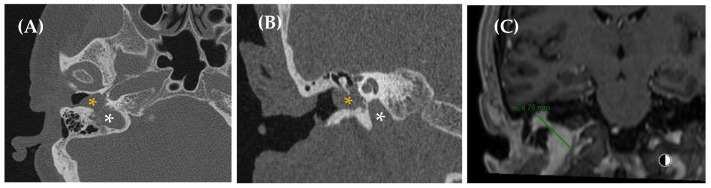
A jugulotympanic paraganglioma in a 60-year-old woman presented with somatosound in the right ear, which increased with exercise and had been present for a long time. The CT scan (axial section, image (A); coronal section, image (B)) revealed a tumor at the level of the jugular bulb (white asterisk) with a tympanic component (orange asterisk). T1-weighted coronal post gadolinium MRI (image (C)) showed a hypercapillary lesion in the jugular bulb on the right side, extending into the tympanic cavity with avid enhancement (green line). The component of the lesion located in the jugular bulb measures around 18 × 12 mm and, in the tympanic cavity, 10 × 14 mm, suggesting a diagnosis of right jugulotympanic paraganglioma.
